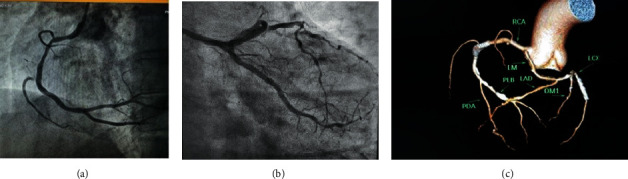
(a) Post-PCI image of RCA showing coronary spasm just proximal to RCA stent after postdilatation. (b) Post-PCI angiogram of LCX. (c) Coronary computed tomography angiography done prior to discharge, delineating the origin and the course of the anomalous artery and patent stents. PCI: percutaneous coronary interventions; RCA: right coronary artery; LCX: left circumflex artery.
